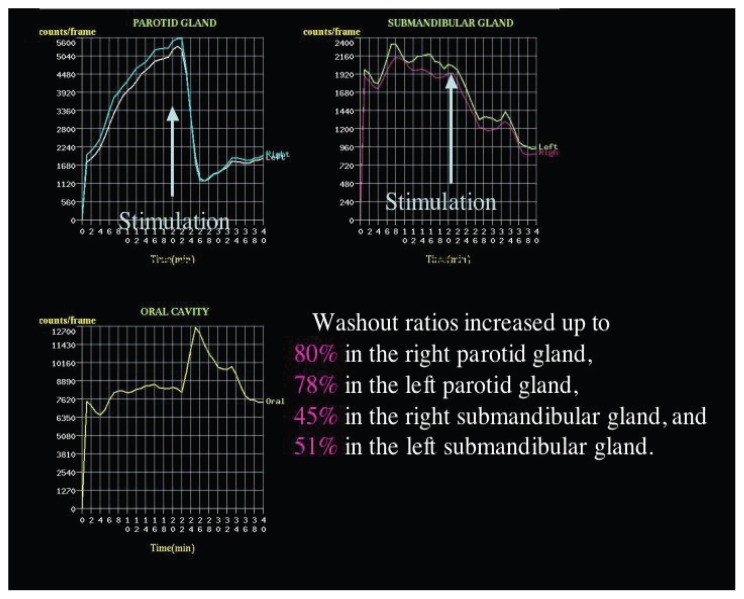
Saliva scintigraphy after treatment with nizatidine demonstrated the increased washout ratios in all four major glands.
